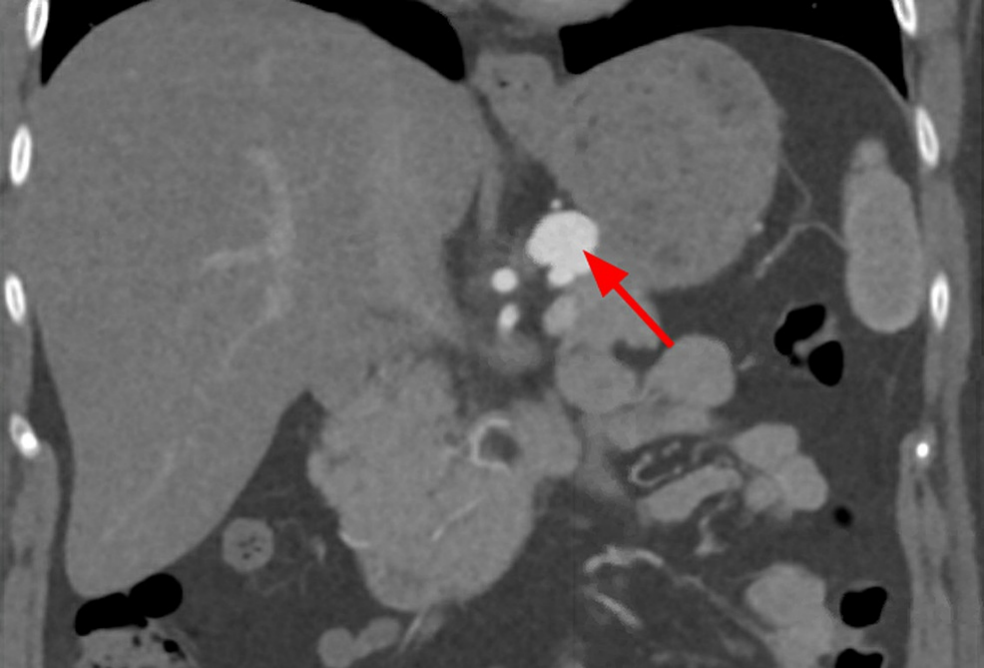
Coronal view of CTA abdomen and pelvis demonstrating the filling of contrast in the splenic artery due to an aneurysm (red arrow). CTA, computed tomography angiography
